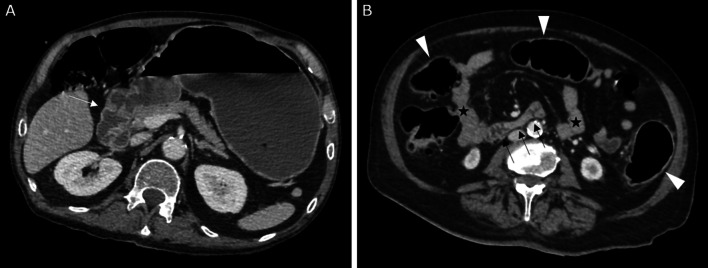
Gastroparesis. Axial CT images (portal venous phase) at the level of the pyloroduodenal junction (A) and of the third part of the duodenum (B), showing a marked gastric dilatation upstream of the pyloroduodenal junction (white arrow), without any mechanical obstruction. There is no duodenal dilatation (black arrows), neither of the other parts of the small intestine (black stars). Note the mild aeric colic distention (black arrowheads)
